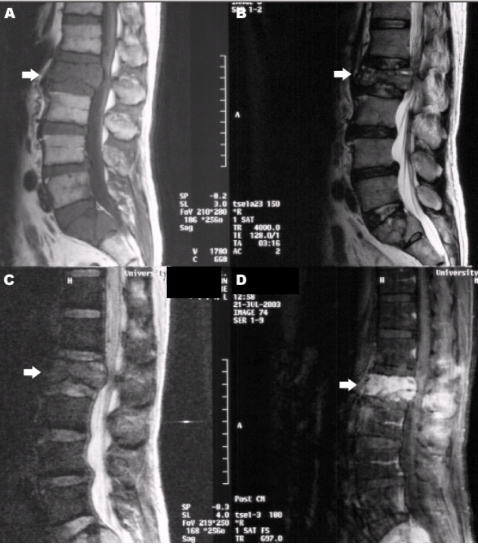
MR images of a patient who presented with compression fracture of L2 vertebral body. There are low signals on both T1 (A) and T2 (B)-weighted MR images, with marked contrast enhancement (D) of L2 and S1 vertebral bodies. SSFP DW MR image shows high signal in the compressed L2 (arrow) as well as in S1 vertebral bodies suggestive of malignancy. Further investigation revealed a bronchogenic carcinoma.
